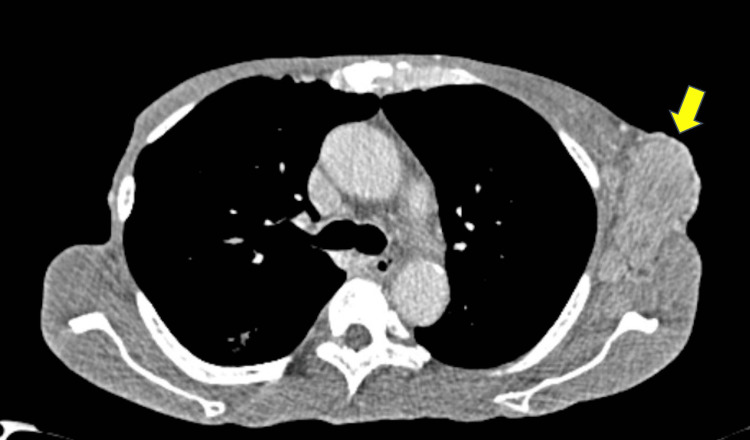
Computed tomography image shows an enlarged left axillary lymph node measuring 6x3 cm (yellow arrowhead)
